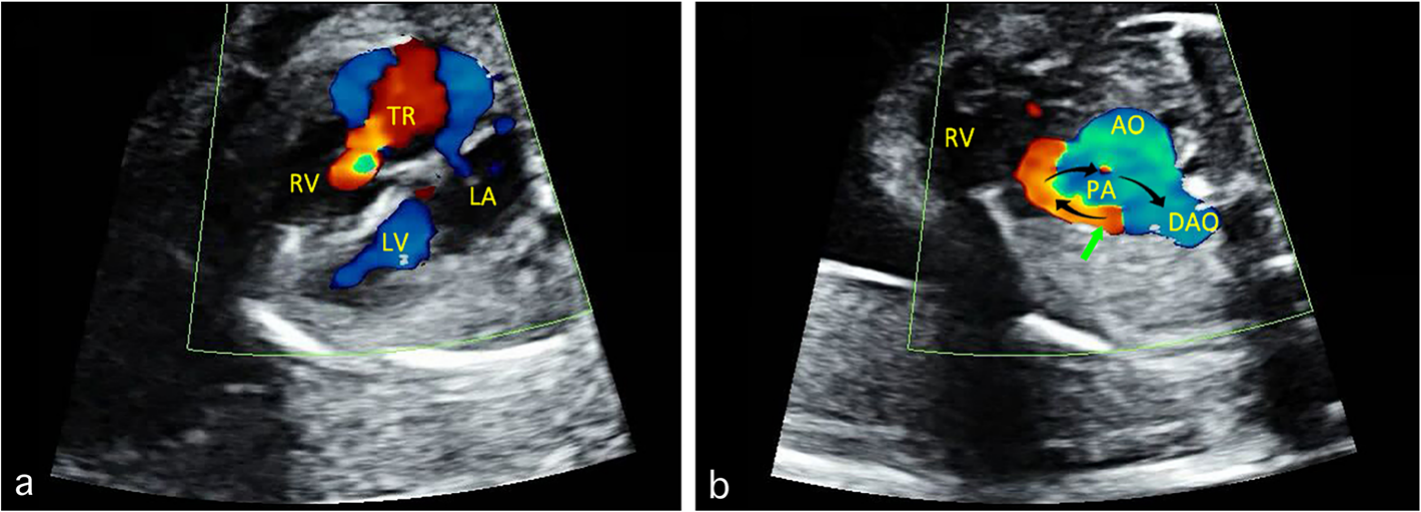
Sonograms showing fetal pulmonary valve atresia. The four-chamber view showed RA dilatation, hypertrophic RV, and severe TR (a). The three-vessel trachea view shows that the blood flow direction (indicated by the arrows) in the ductus is from DAO to the PA, instead of the normal PA-DAO direction. In addition, no blood is visualized going through the pulmonary valve (b). AO: aorta; DAO: descending aorta; LA: left atrium; LV: left ventricle; PA: pulmonary artery; RA: right atrium; RV: right ventricle; TR: tricuspid regurgitation
